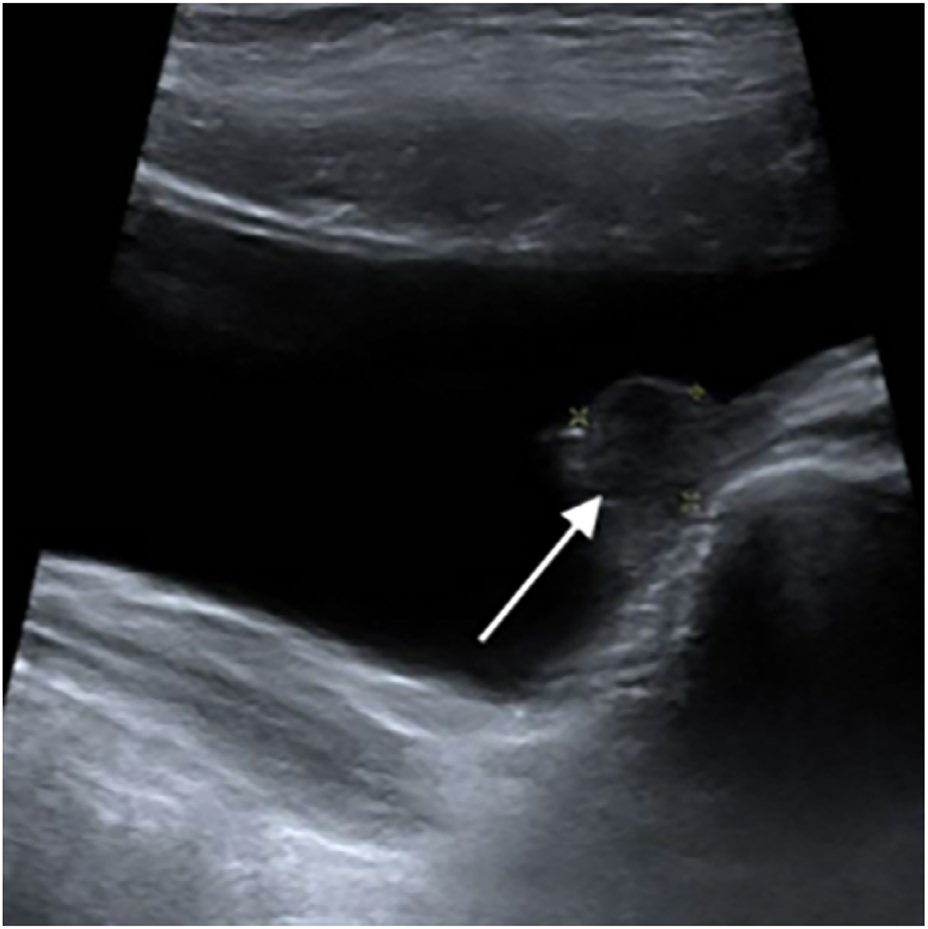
Ultrasonography with a 10 mm nodule at the left posterolateral wall of the bladder (white arrow).
